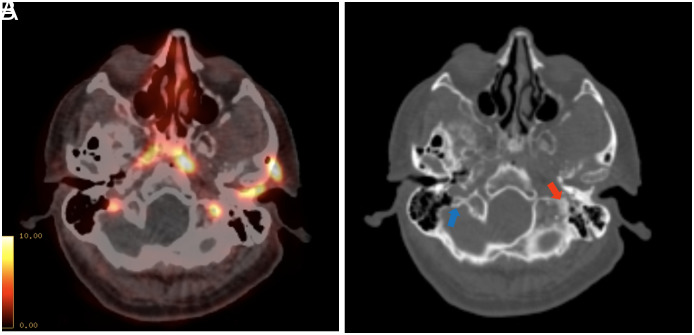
A, B. A 66 year-old patient underwent PSMA PET/CT due to a rise in serum PSA to 3.1 ng/dL post-radical prostatectomy. The PSMA PET scan revealed widespread metastatic disease at LN and multiple bones including left occipital and bilateral sphenoid metastases. (A) Axial fusion image depicting 2 PSMA-expressing metastatic tumors in the right and left sphenoid bones. (B) Standard CT image revealing the metastatic lesion on the right side (SUVmax: 5.63) does not have a CT counterpart while the metastatic lesion at the left sphenoid bone (SUVmax: 14.03) exhibits an osteosclerotic CT counterpart.
